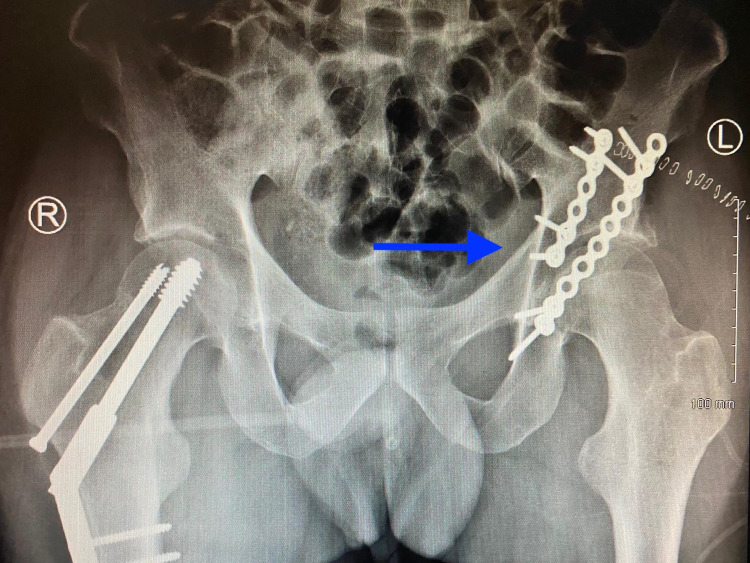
Plain Radiographic Example of a Left Acetabular Fracture Sustained During the COVID-19 Pandemic Requiring Surgical Fixation
